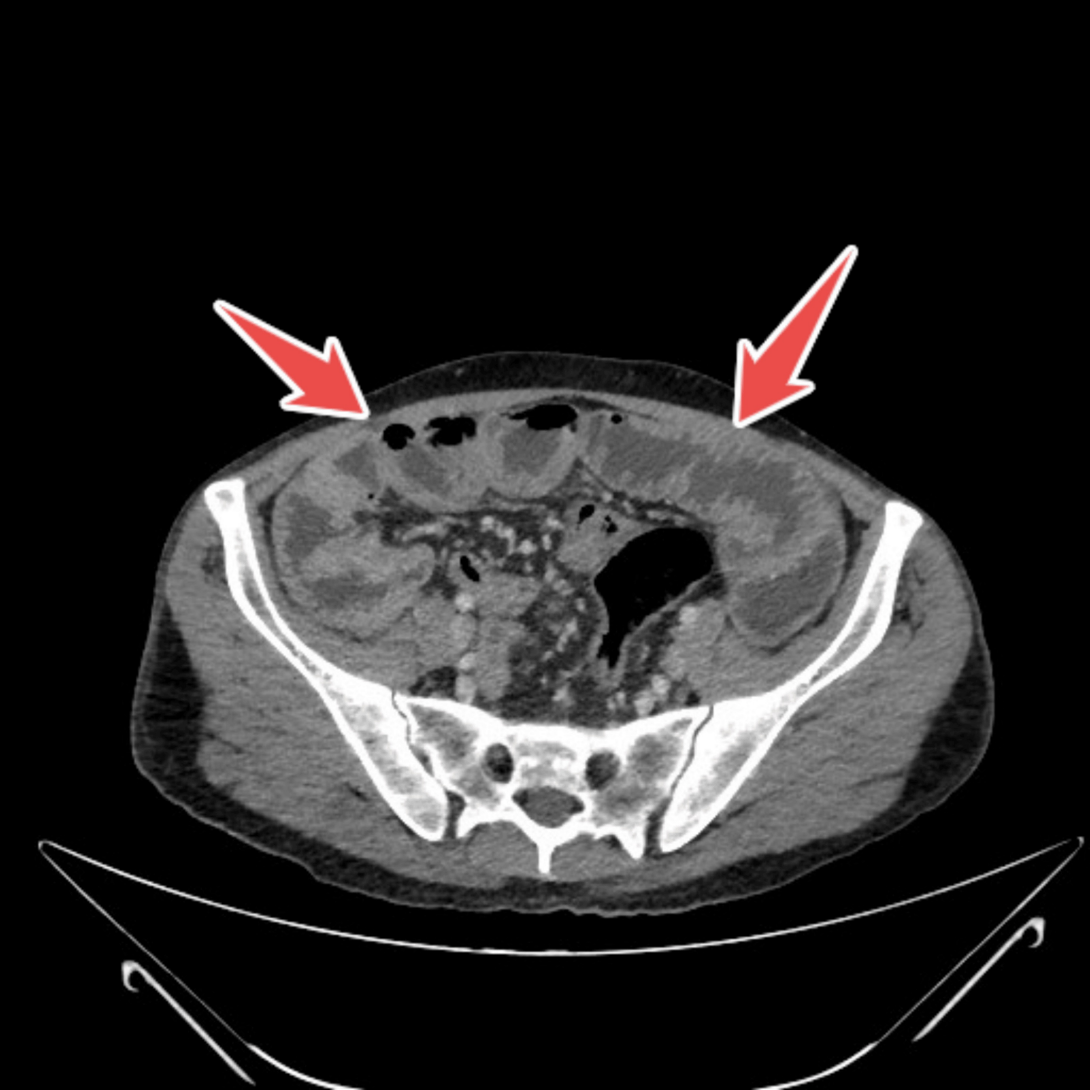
CT abdomino-pelvic showing relative absence of jejunal folds, thickening of the ileum, up to 1 cm in some areas highlighted by arrows
